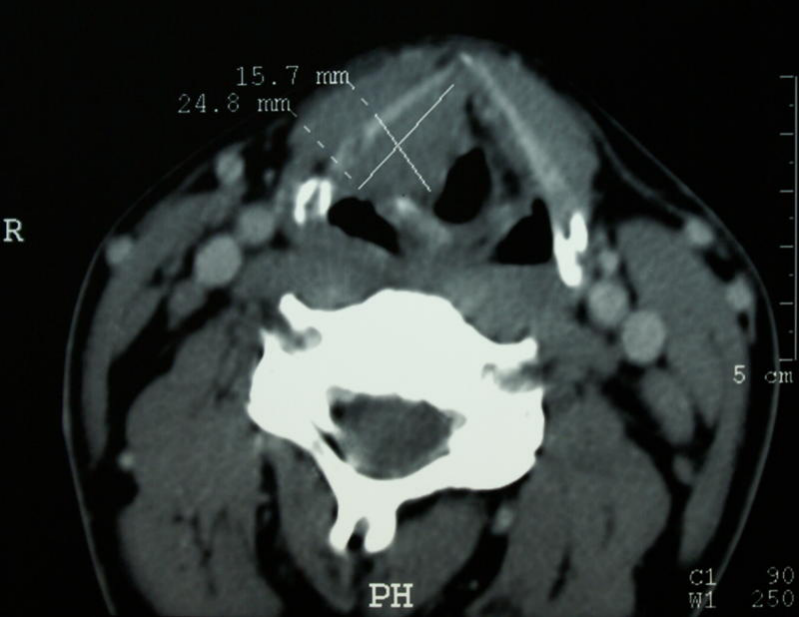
A two-phase CT scan, performed in August 2005 showing a 24.8 × 15.7 mm poorly delineated tumor, located at the right side of the larynx, invading the vocal fold and the ventricular fold, contracting the pyriform recess and constricting the glottis.
